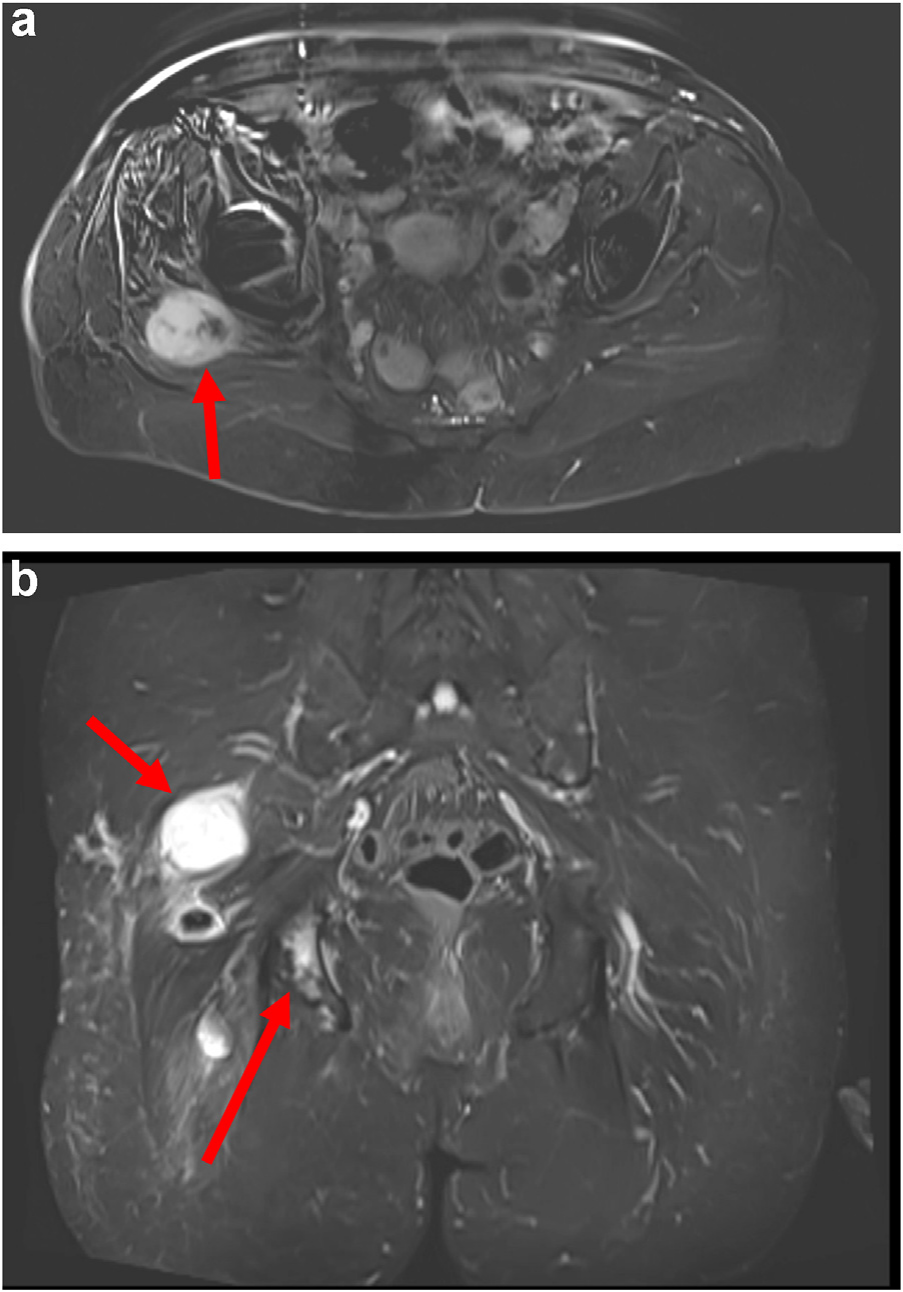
(a) Axial and (b) coronal T2 weighted MRI 5-year status postresection and proximal femoral replacement demonstrating hyperintense soft tissue mass posterior and superior to the right hip in the gluteal musculature as well as hyperintensity of the ipsilateral ischial tuberosity, which was later found to be a biopsy-proven recurrence of synovial sarcoma as well as associated osseous metastasis to the right ischium.
